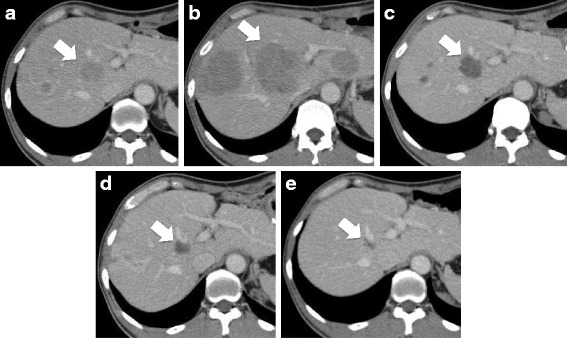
Liver metastases from malignant melanoma which showed early pseudoprogression during treatment with nivolumab. a Liver metastases (arrowhead indicates the target lesion: 31 mm) before the start of treatment with nivolumab. b CT at the 3-month assessment: the liver metastases had increased in size (target lesion: 63 mm). c CT at the 5-month assessment: the liver metastases had shrunk (target lesion: 31 mm), associated with a change of the density. d CT at the 8-month assessment: the target lesion was 20 mm in diameter. e CT at the 12-month assessment: the target lesion was 13 mm in diameter
